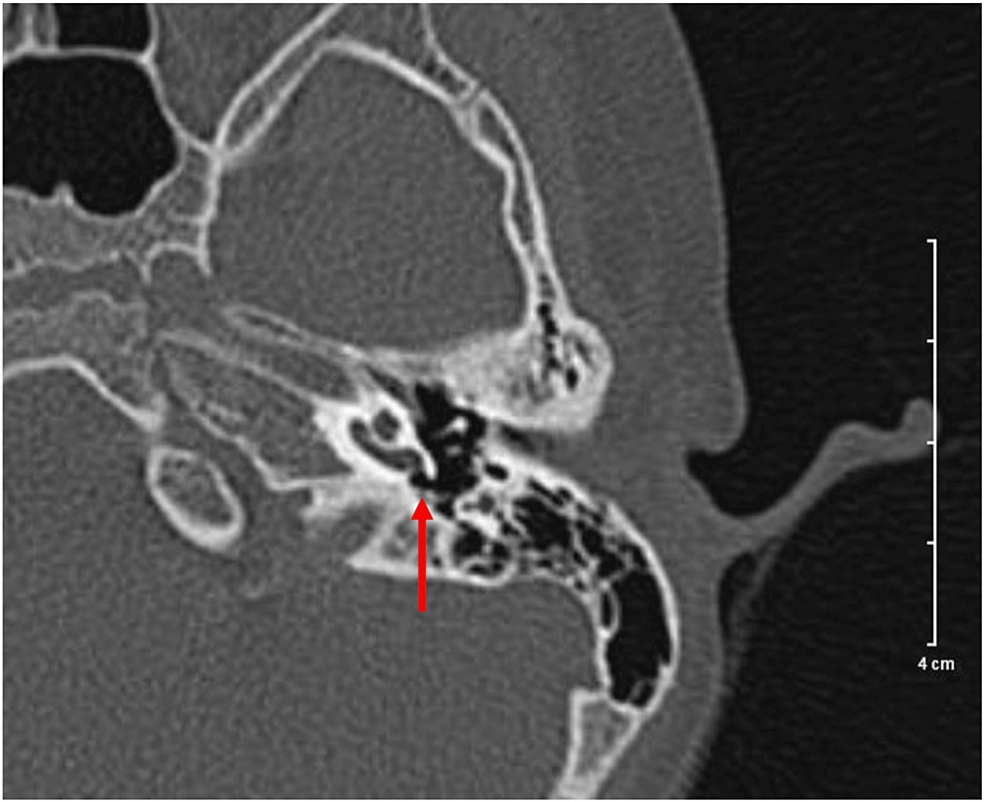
High-resolution computed tomography (HRCT) of temporal bone showed the presence of round window membrane over the left ear as depicted by the red arrow.
